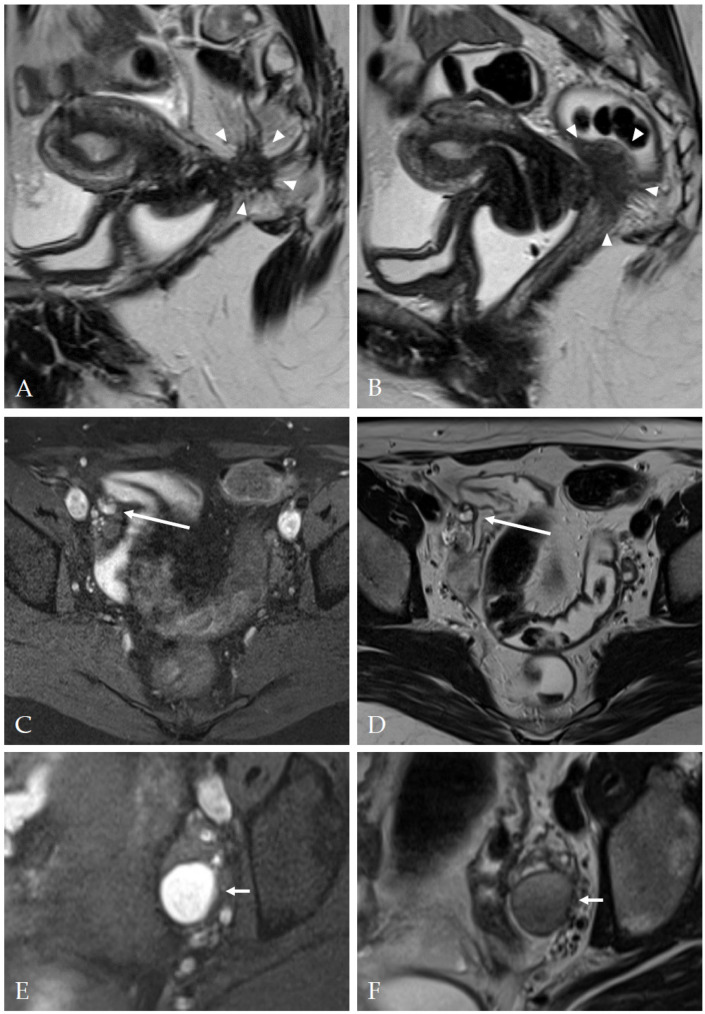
Magnetic resonance imaging examples of deep infiltrating endometriosis and endometriomas. (A,B) Two slices of a sagittal T2-weighted FSE (fast spin echo) sequence, depicting a mostly hypointense mass with ill-defined margins and hyperintense foci (arrowheads) invading the vaginal vault and the rectum (Enzian A2, B3, C3, DEMS (deep infiltrating endometriosis morphology score) 3). (C) Axial T1 FSE with fat suppression and (D) axial T2 FSE sequence showing a nodule with T1- and T2-hyperintense foci at the right pelvic sidewall (long arrow) (Enzian B1, DEMS 3). (E) Axial T1 FSE with fat suppression and (F) axial T2 TSE showing a typical endometrioma (short arrows, T1-hyperintense, T2-hypointense with ‘shading’) (Enzian O2).
